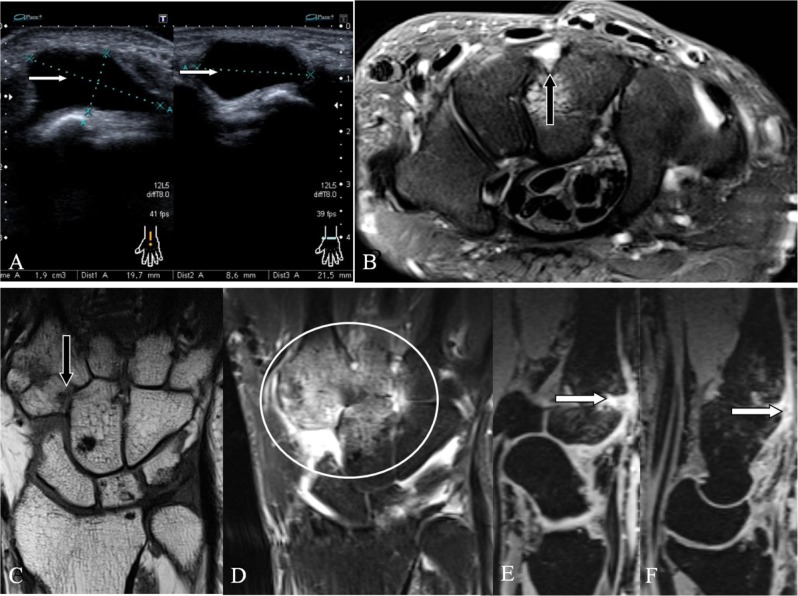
CB due to a soft tissue lesion. (A–B) A dorsal ganglion cyst (arrows) in two different patients. (A) Ultrasound, (B) Axial SE PD-WI FS. Protuberance at the CMC joint due to a large (A) and a small (B) dorsal ganglioncyst (arrows), both asymptomatic. (C–F) Contrast enhancement in CB due to rheumatoid arthritis. (C) Coronal SE T1-WI, (D) Coronal SE T1-WI FS with gadolinium contrast, (E–F) Sagittal 3D-GRE. (C) Arthritis with erosion at the quadrangular joint (arrow). (D) Prominent contrast enhancement around the quadrangular joint (oval). (E–F) Dorsal prominent synovitis (arrows) at the (E) second and (F) third CMC joint.
